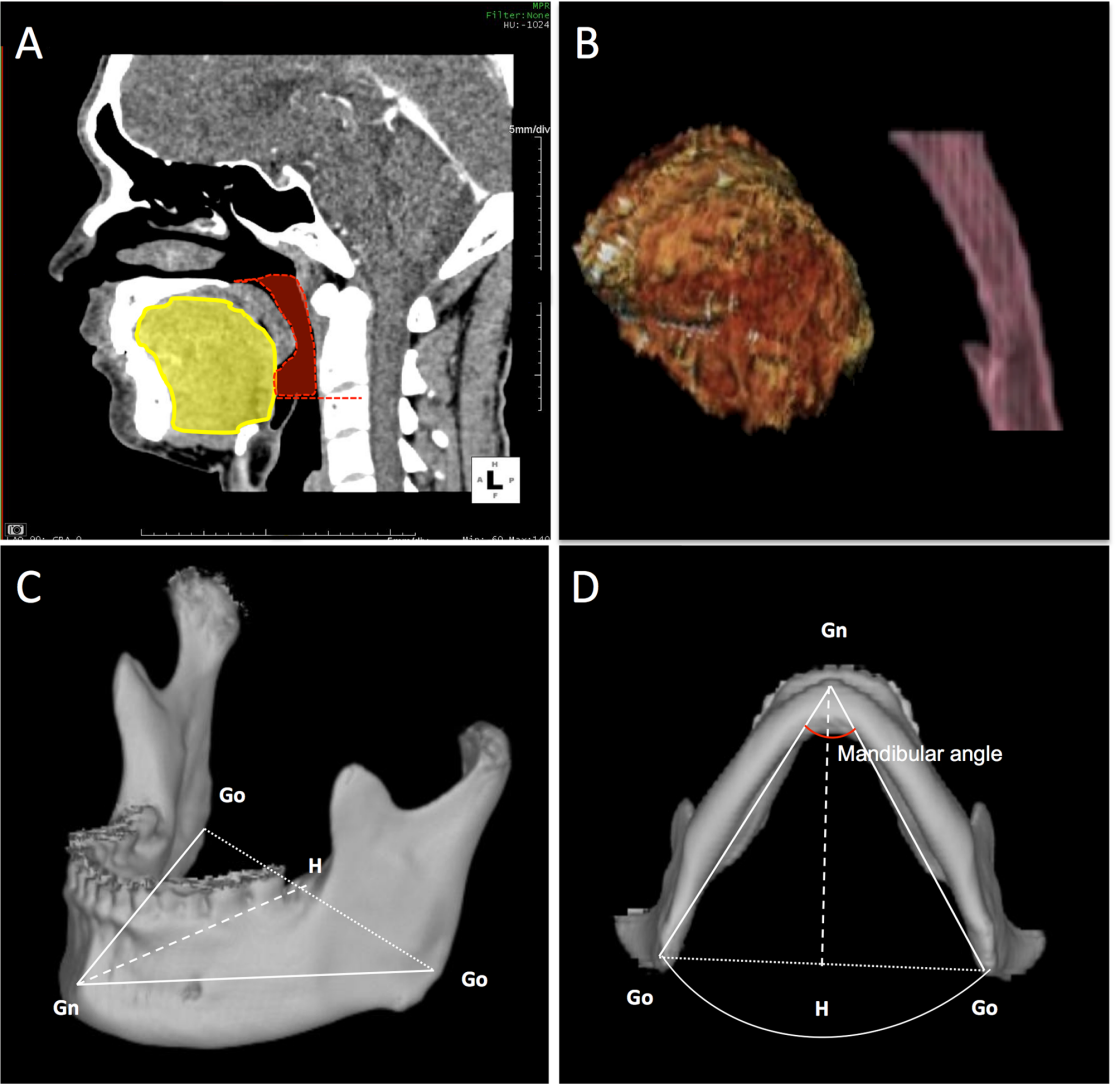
Analysis of three-dimensional (3-D)-reconstructed computer tomography (CT) images. A. The tongue (yellow) and posterior airway space (red) are seen on a sagittal reformatted image. B. Three-dimensional segmentation of the tongue and posterior airway space. C. Oblique view of the reconstructed mandible, which is marked with measured points. D. Inferior view of the reconstructed mandible showing intra-mandibular area. Gn; gnathion, Go; gonion, H; midpoint between both gonions, Gn-H; mandibular depth.
